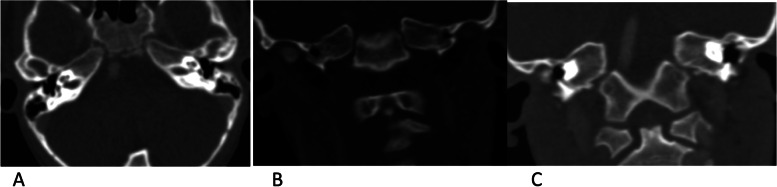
Absence of bony carotid canals in petrous bones detecting with bone window axial (A) and coronal (B and C) CT images, confirming the finding of agenesis both ICA
